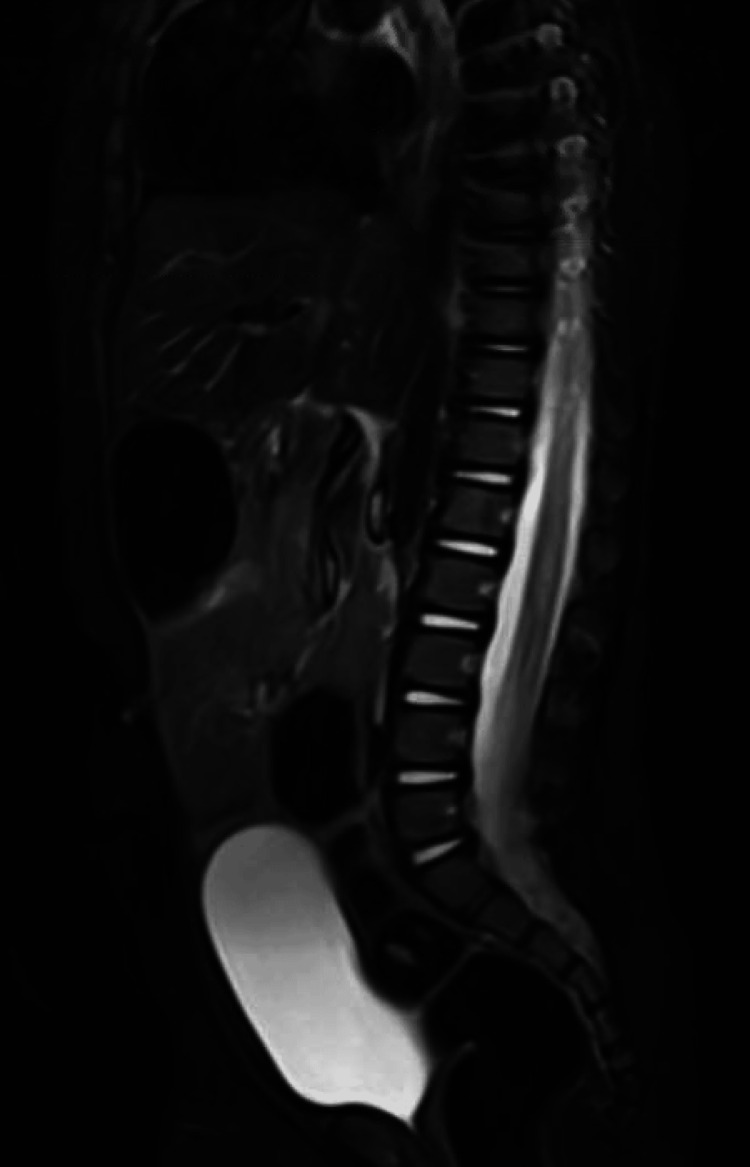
Spinal section of initial whole-body T2-weighted short tau inversion recovery MRI (sagittal view) demonstrating no abnormal findings With no abnormalities seen at the L5–S4 levels, this highlights the limitation of whole-body MRI in detecting subtle spinal pathology such as a dermal sinus tract.
